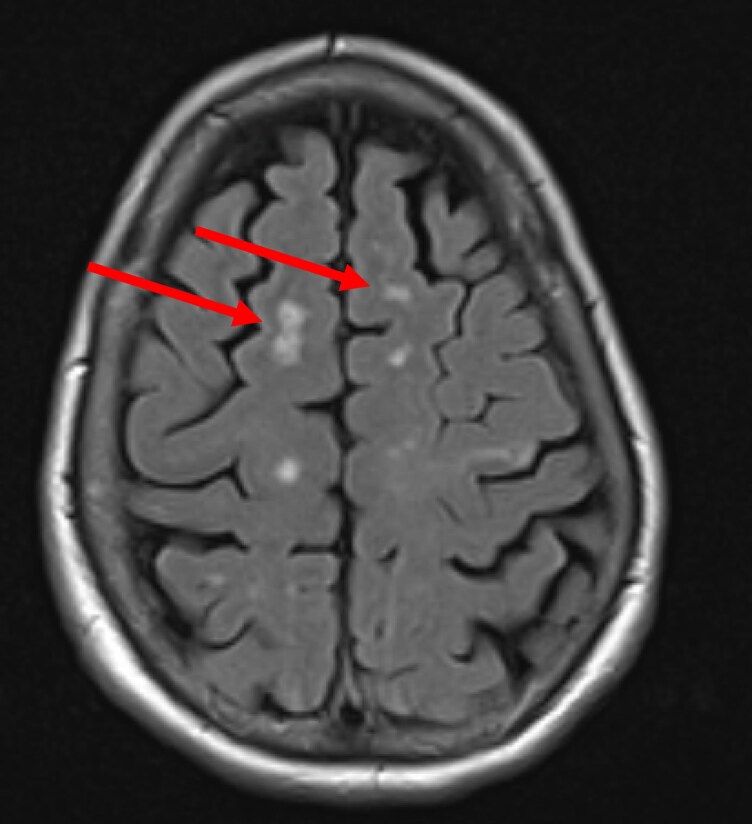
Brain MRI showing multiple bilateral lacunar stroke (arrow).
